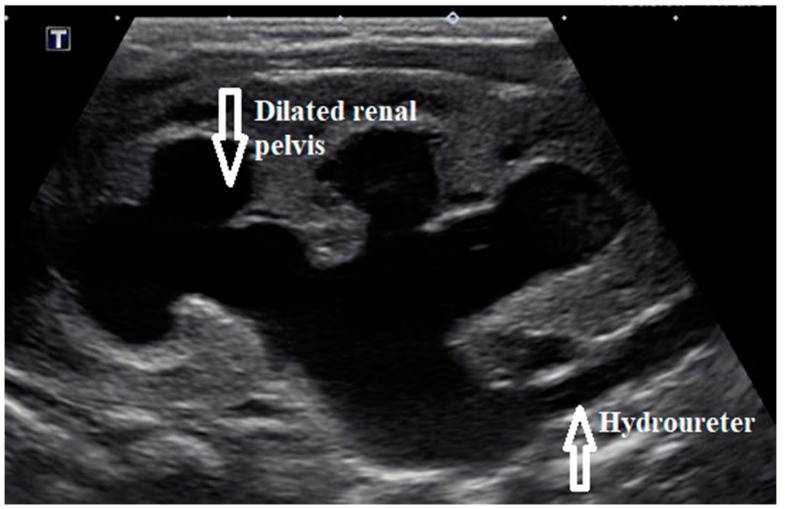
Left renal sonogram showing severe hydronephrosis and hydroureter.
